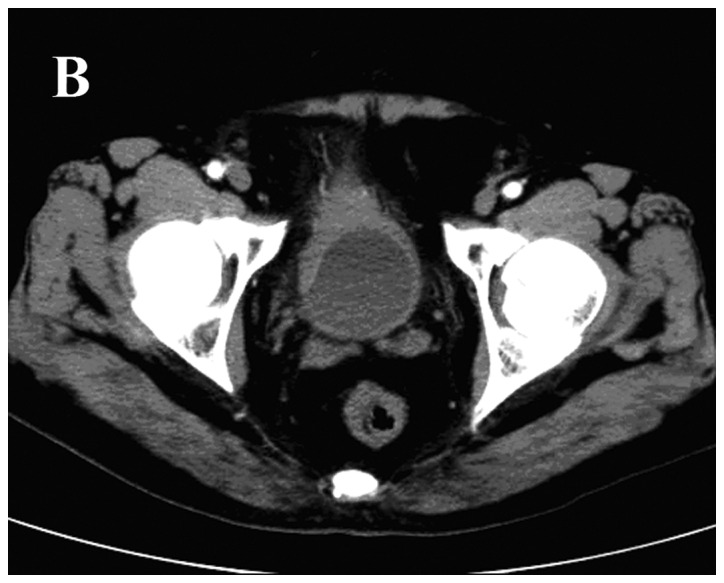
Radiological features of the case. Arterial-phase computed tomography scan revealing (A) a 2.5 cm-diameter solid pedunculated mass at the right side of the bladder wall, and (B) thickened anterior and right bladder walls with significant mucous membrane enhancement. No significant bladder mass was detected.
